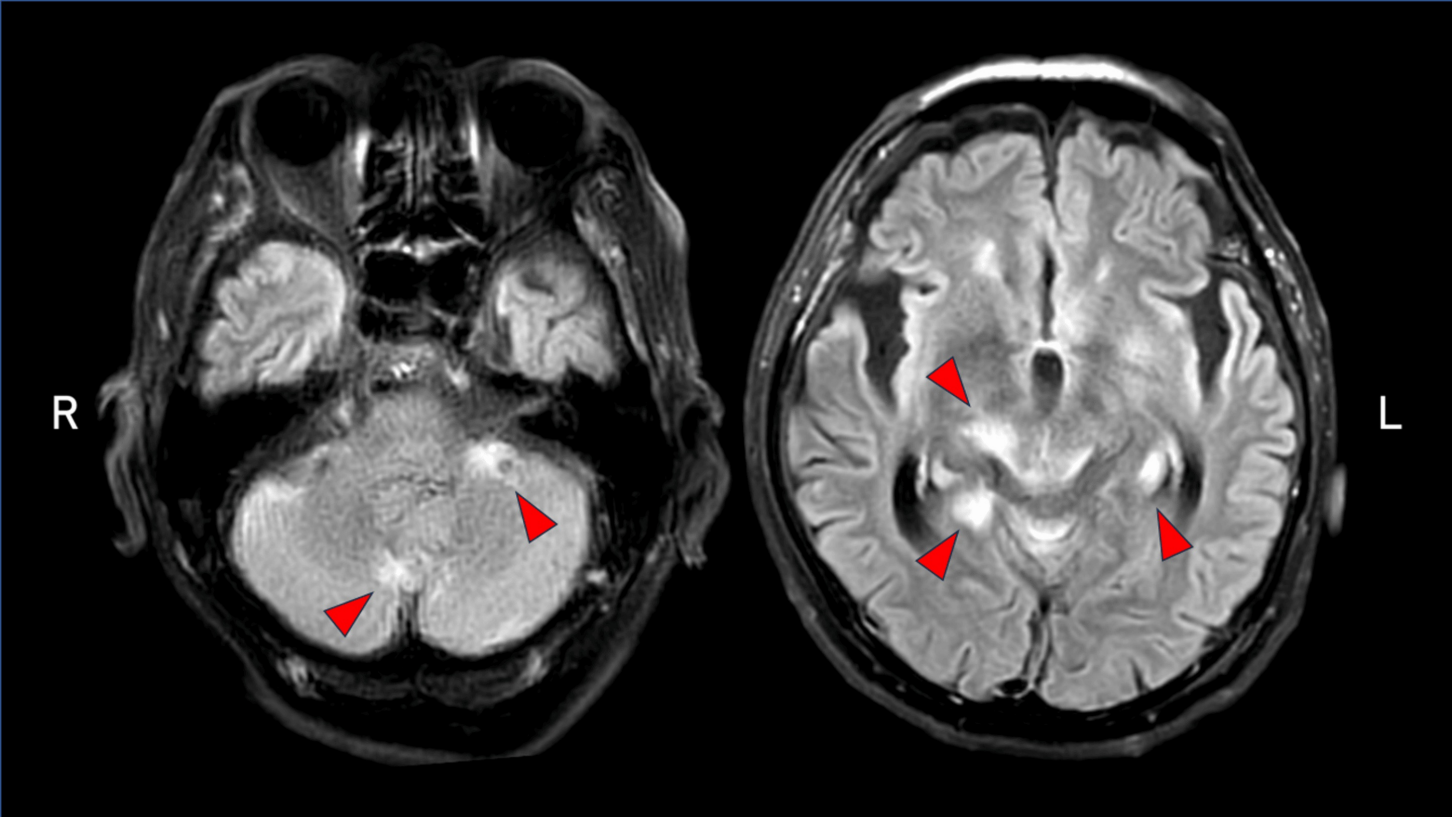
Fluid-attenuated inversion recovery axial MRI showing multiple high-intensity lesions in the cerebellum, periventricular, and hippocampus.
